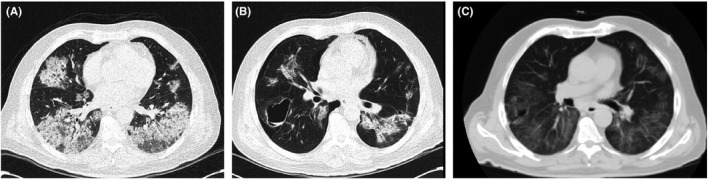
Chest CT scans of case 3
